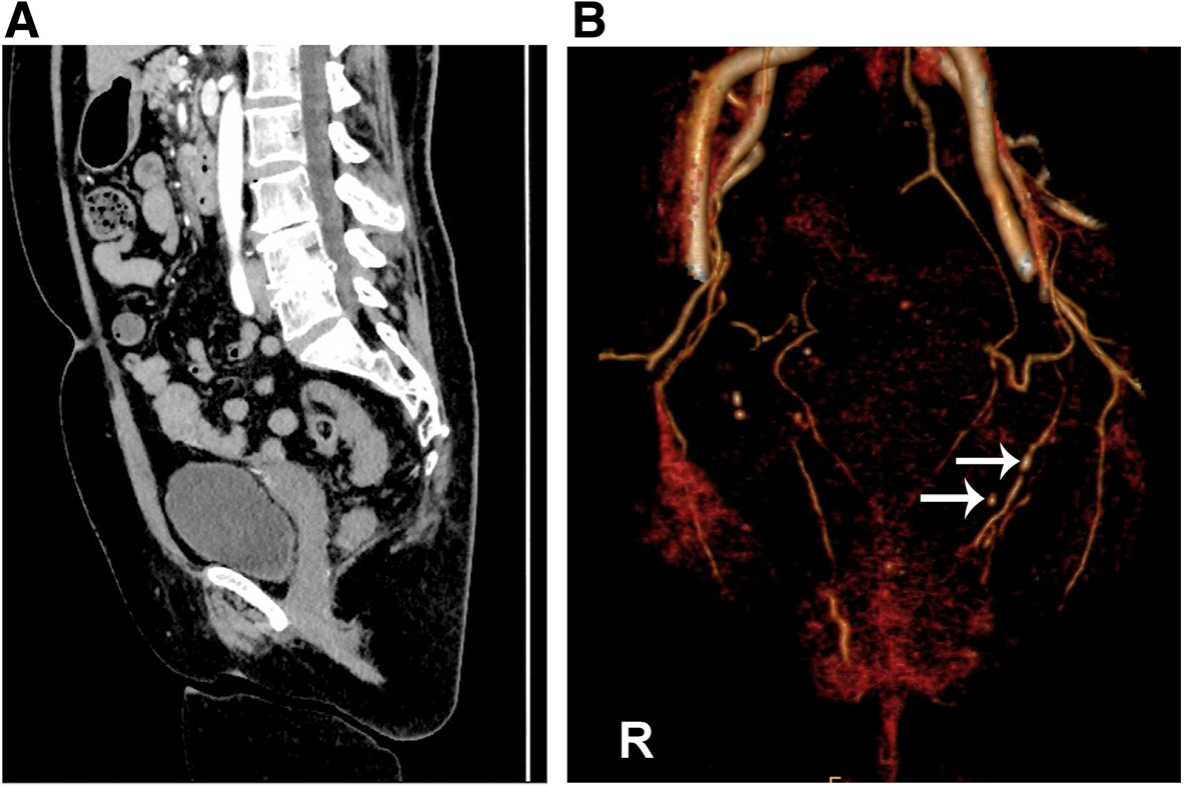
Lesions in the pelvic cavity. a Contrast-enhanced computed tomography (CT) scan of the abdomen and pelvic cavity. b 3-D reconstruction of the pelvic vessels. White arrows: enlarged vein and varicosities in the left pelvic region. R: right side
